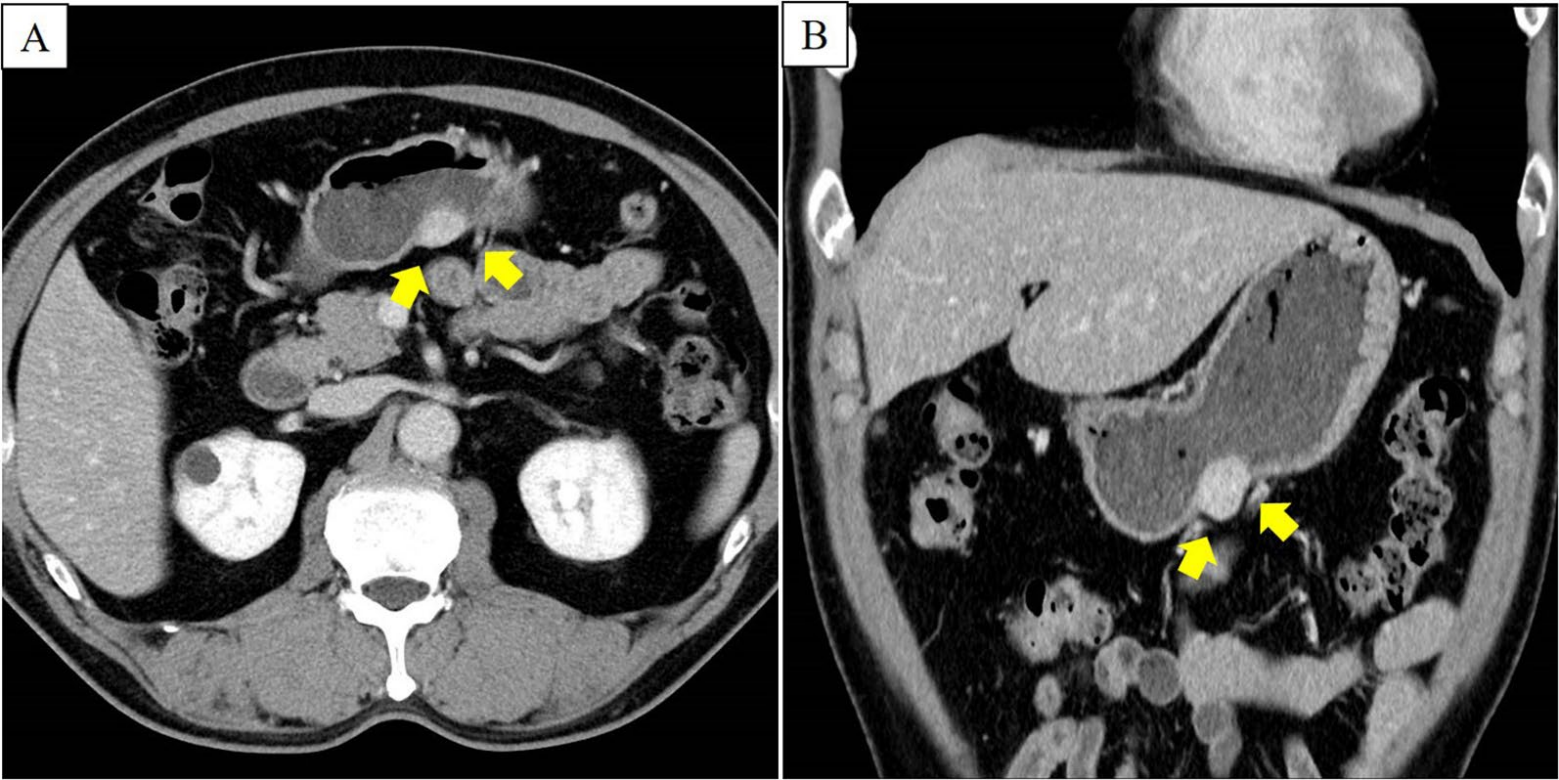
Abdominal enhanced computed tomography. A Horizontal view. B Coronal view. Abdominal enhanced CT scan showed a contrast-enhanced round mass on the posterior wall of the stomach (yellow arrows), with no evidence of tumor metastasis
